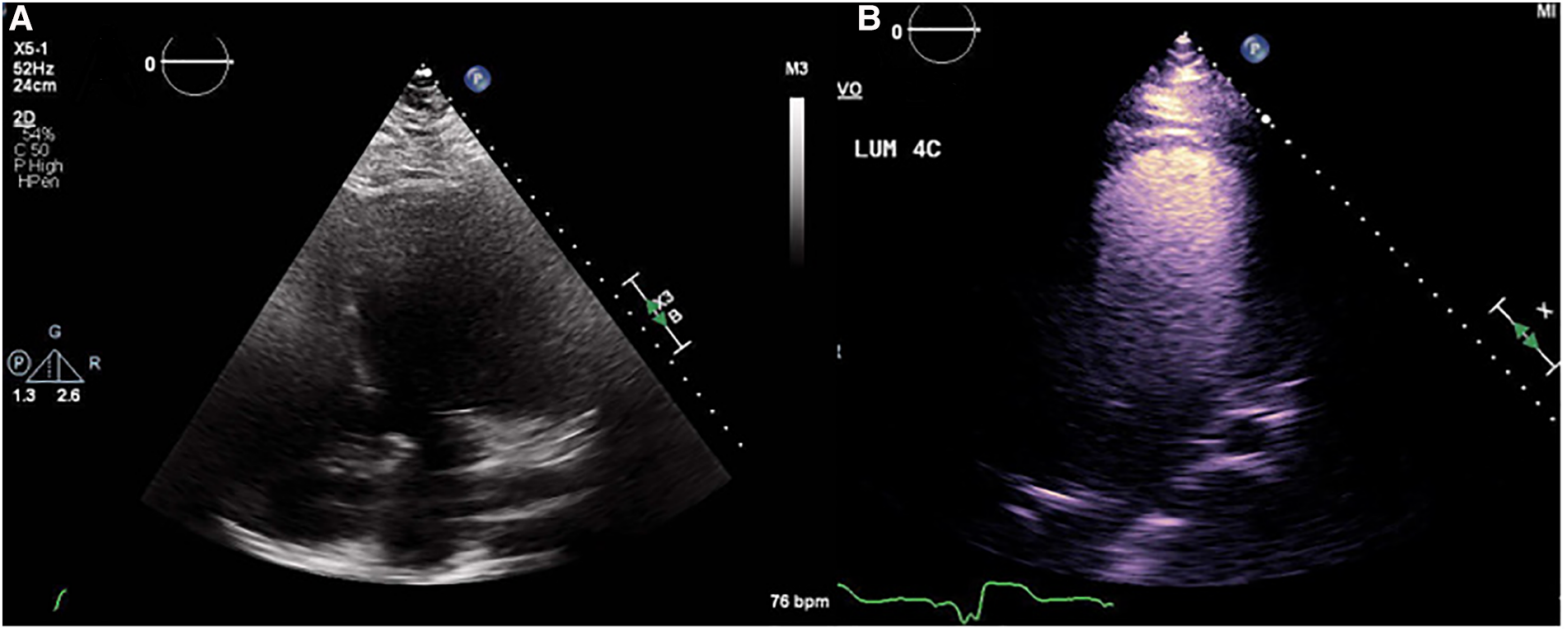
(A) Non contrast echocardiograph beside (B) contrast echocardiograph depicting the improvement in structure visualization.
